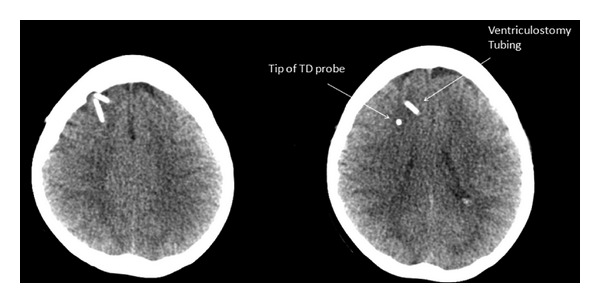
Postoperative head CT scan demonstrating the position of the thermal diffusion blood flow probe.
